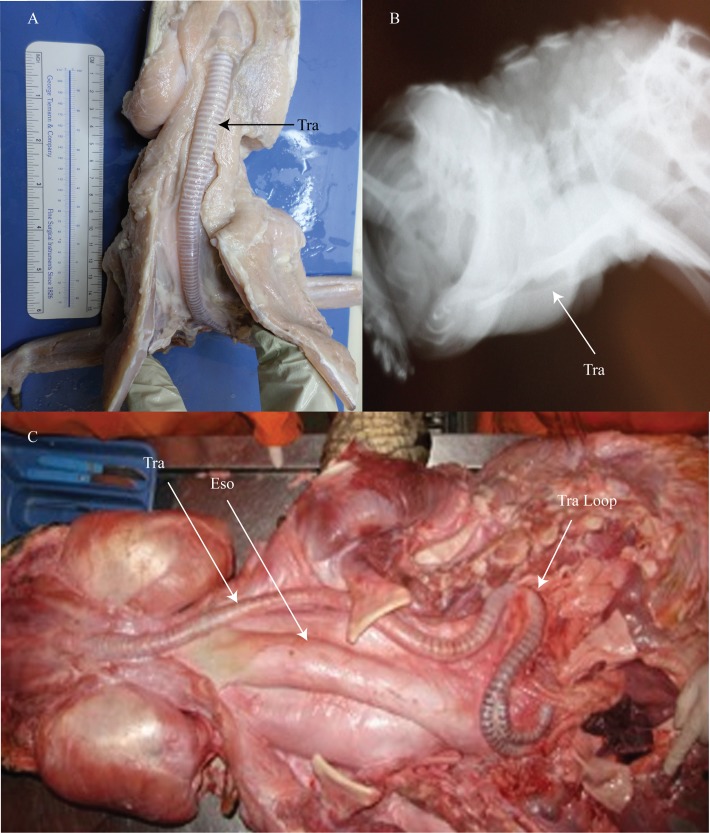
Alligator mississippiensis* and *Crocodylus niloticus*. (A) ventral view of *A*. *mississippiensis* trachea. (B) radiograph of alligator trachea. (C) ventral view of the Nile crocodile’s trachea and esophageal course. Tra = trachea; Eso = esophagus; Tra loop = tracheal loop. Nile crocodile image courtesy of J. Reidenberg.
